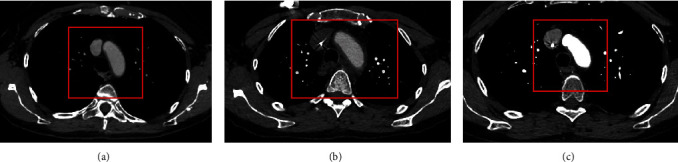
(a) CT chest with contrast of the patient on 2/11/21. (b) CT chest with contrast of the patient on 5/03/21. (c) CT chest with contrast of the patient on 7/01/21. Between February and March 2021, this patient received both pembrolizumab and pegfilgrastim. Her May CT was significant for wall thickening and inflammatory changes surrounding the left subclavian artery extending into the aortic arch with involvement of the left carotid artery and proximal innominate arteries, new findings as compared to her February CT. After the initiation of a long steroid taper in May, the patient's CT chest in July showed marked improvement of the wall thickening and inflammatory changes of the aortic arch and arch vessels, most compatible with resolving vasculitis.
